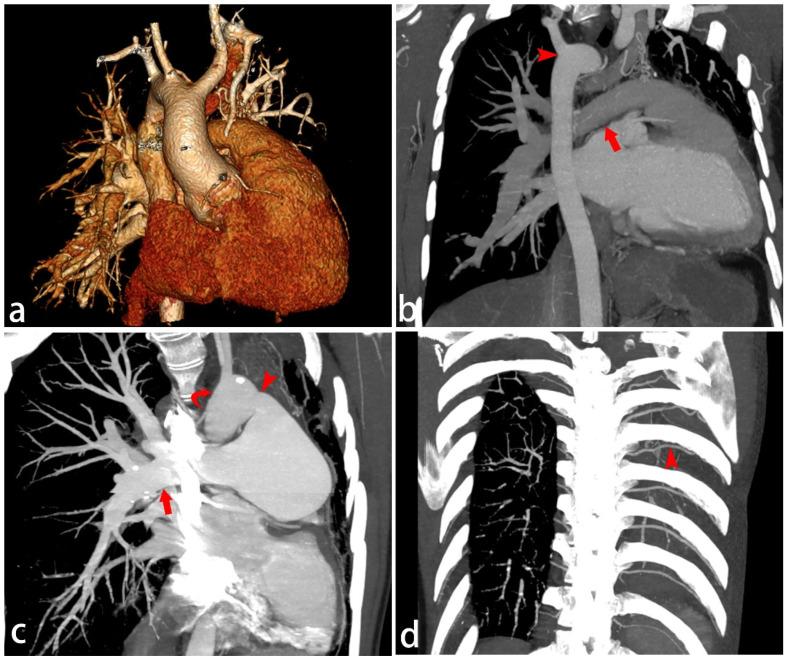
(a,b) A 15-year-old male with proximal interruption of the left pulmonary artery. Volume-rendered CT angiography (a) and maximum intensity projection (MIP) image of CT angiography (b) show the right-sided aortic arch ((b), red arrowhead). The right pulmonary artery is normal ((b), red arrow). (c,d) A 17-year-old female with proximal interruption of the left pulmonary artery. The aortic arch is left-sided ((c), red curved arrow). The right pulmonary artery is in the normal position ((c), red arrow) and there is an associated patent ductus arteriosus ((c), red arrowhead). The left intercostal arteries are dilated, indicating systemic collateral arteries formation ((d), red arrowhead).
